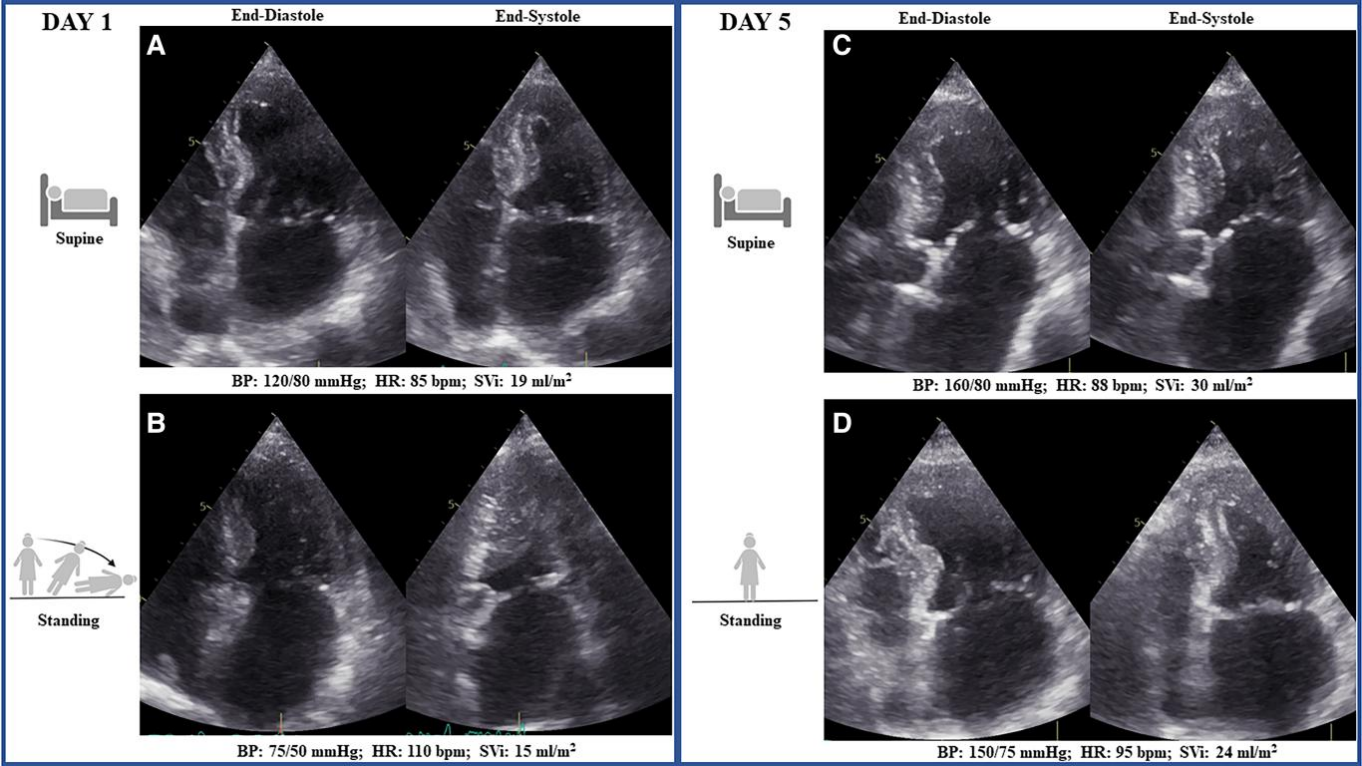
(*A*) At baseline, an echocardiogram in the supine position showed a non-dilated, hypertrophied, small left ventricle with restrictive physiology and reduced stroke volume index (SVi). (*B*) While standing, hypotension and symptoms developed with echocardiographic evidence of mid-left ventricular obstruction (MVO). (*C*) On the fifth day in the supine position, SVi was increased. (*D*) In the standing position, MVO was not present any further. BP, blood pressure; HR, heart rate; SVi, stroke volume index; MVO, mid-left ventricular obstruction.
